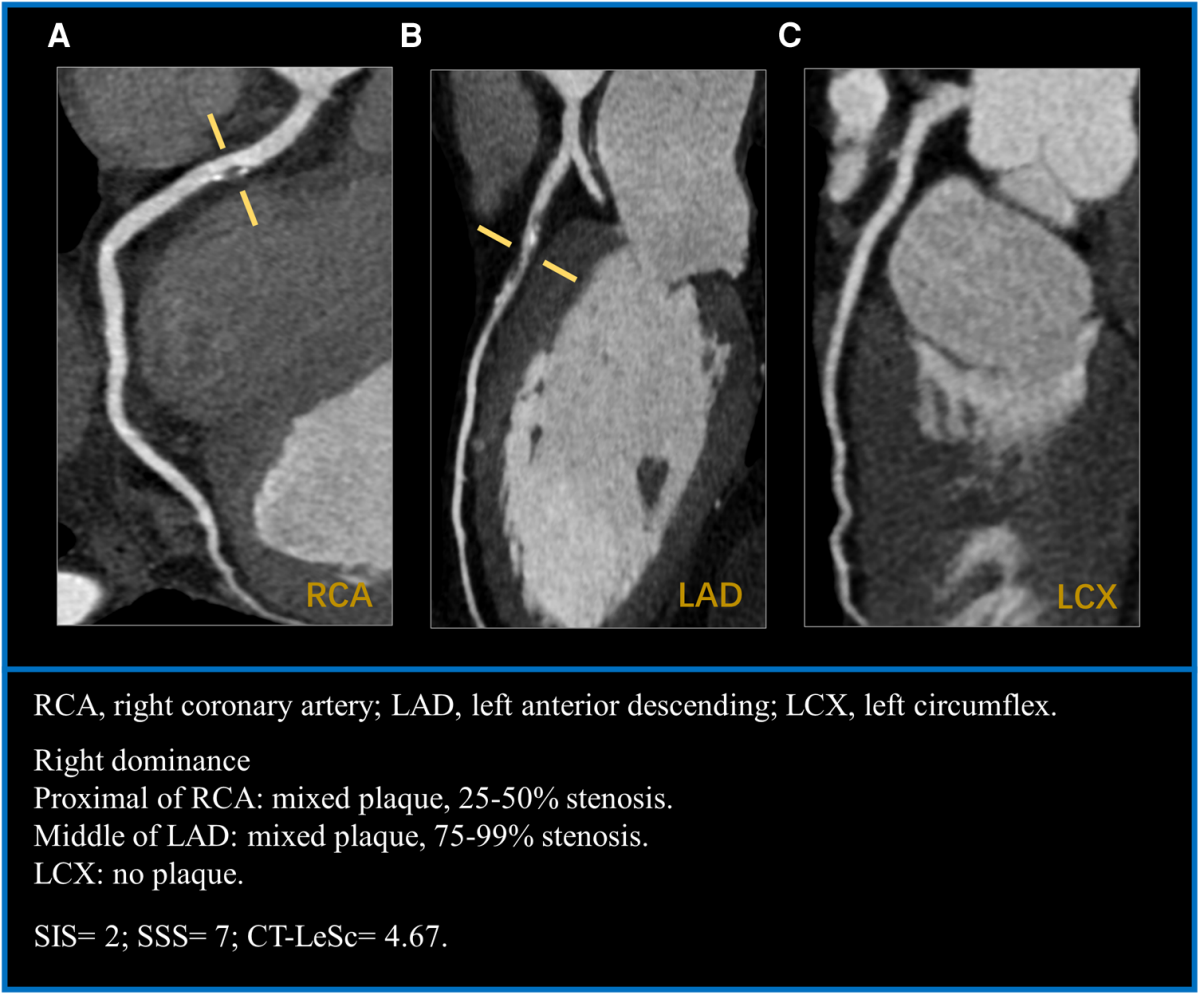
A case example of a 57-years-old man with hypertension for 20 years. (A) mixed plaque with mild stenosis in the proximal segment of RCA. (B) mixed plaque with severe stenosis in the middle segment of LAD. (C) LCX without plaque. CT-LeSc = RCA (1 × 1.5 × 0.615) + LAD (2.5 × 1.5 × 1) = 4.67. RCA, right coronary artery; LAD, left anterior descending; LCX, left circumflex; CT-LeSc, CT-adapted leamanscore.
